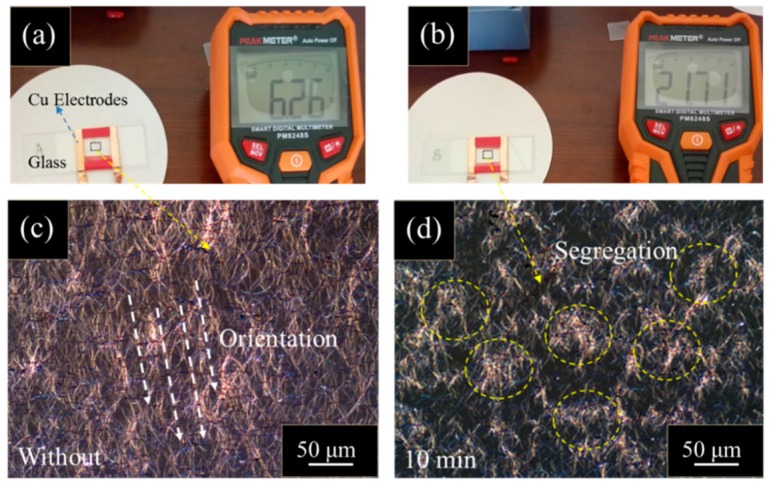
(a,b) Resistivity test of Ag nanowire films without and with 10 min ultrasonic treatment on glass substrates, respectively; (c,d) optic images of the Ag nanowire films without and with 10 min ultrasonic treatment, respectively. The agglomerations of Ag nanowires are marked by yellow dashed circles.
